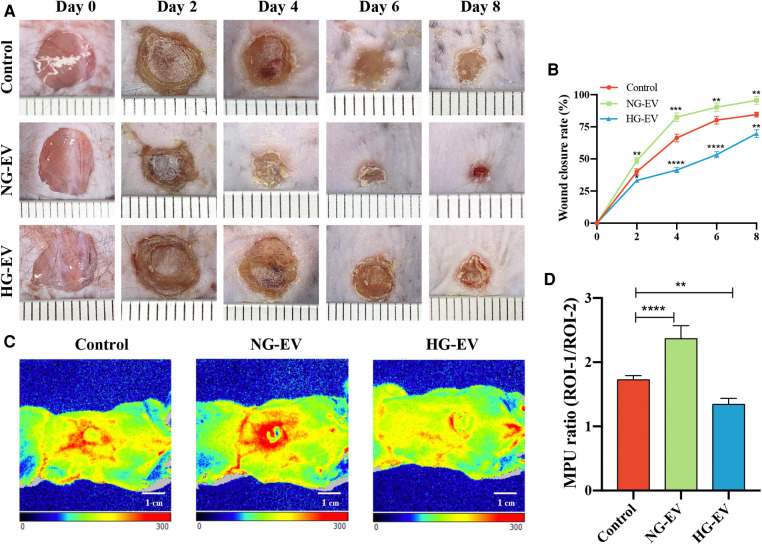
The impact of EVs on cutaneous wound healing and blood perfusion. (A) Healing progression of the wound from day 0 to day 8. (B) The statistical analysis of wound closure area in each group (*n* = 5). (C) The blood flow at the wound site in each group that was evaluated by laser Doppler perfusion imaging system. (D) Quantitative perfusion intensity of the wound area in the three groups.
